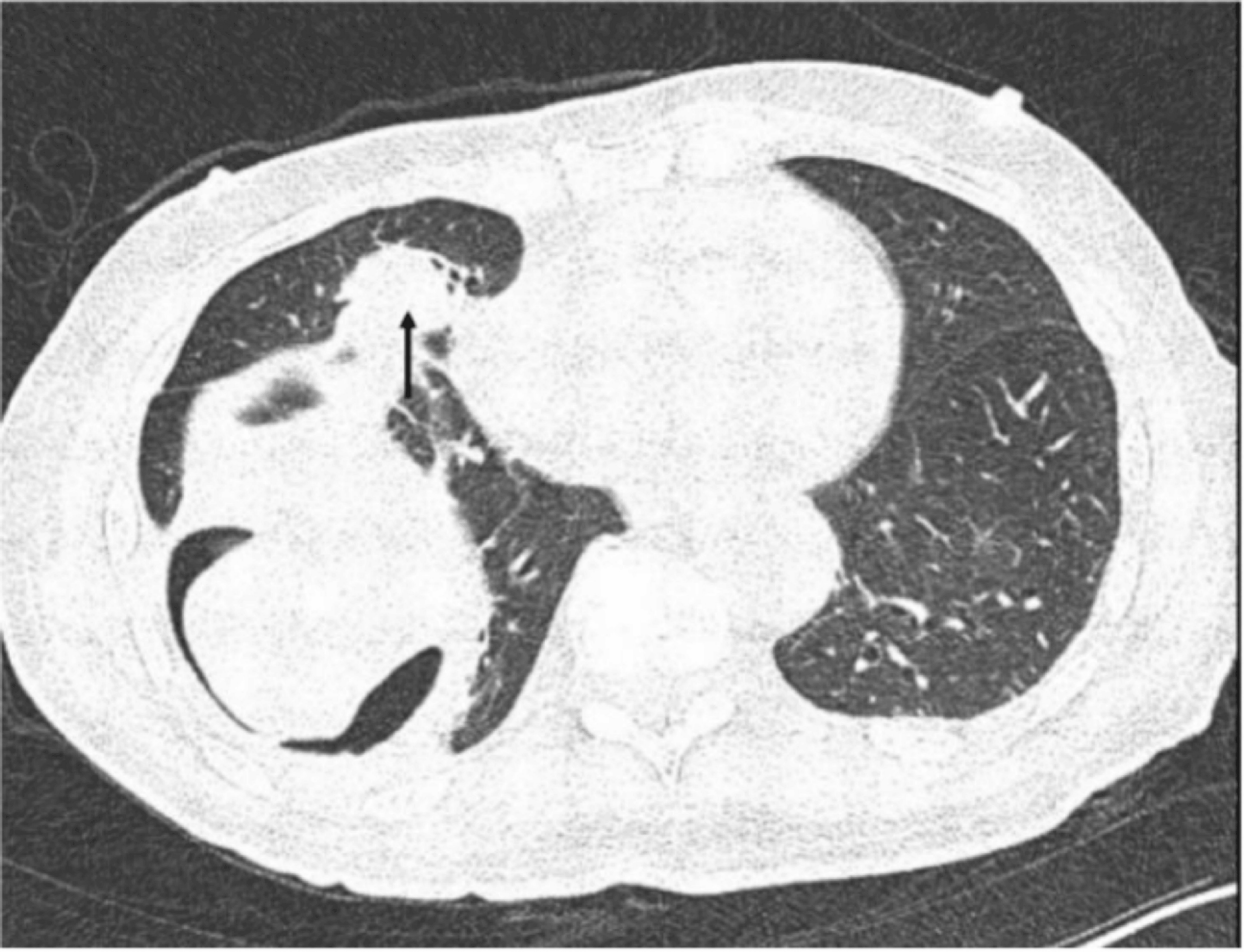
Axial view of CT chest (lung window) with right-sided diaphragmatic lesion (black arrow)
